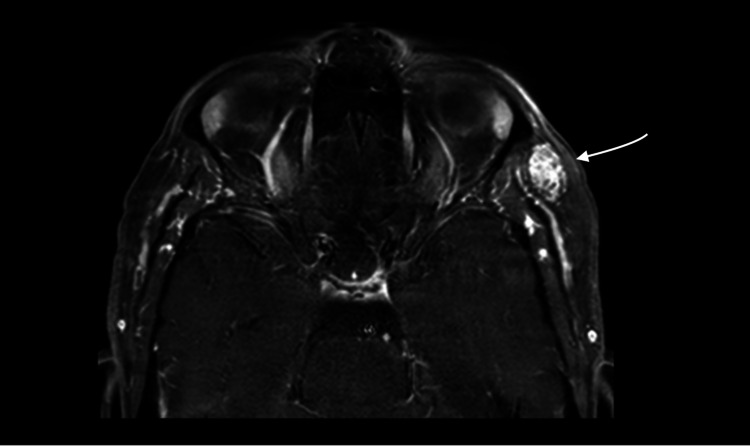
MRI with contrast imaging, axial view The arrow shows the identified lesion
